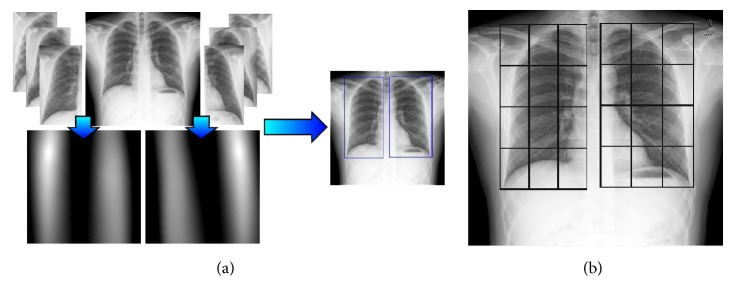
Lung location and segmentation in a radiograph. (a) Application of template matching to a radiography (left and right patterns), matching maps, and the obtained optimal location. (b) The detected lungs are divided into two grids of regions.
